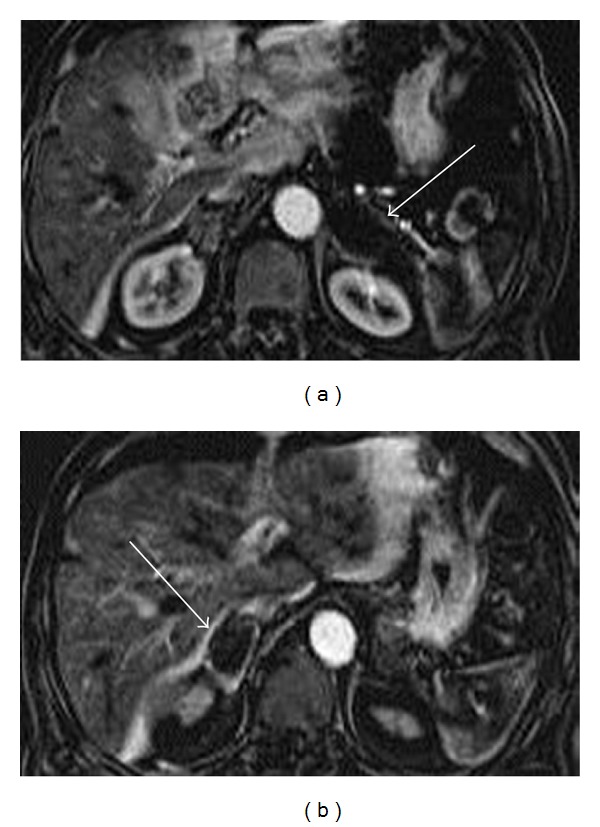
On axial contrast-enhanced subtracted MR images (a), (b) any signal from blood products is removed and the hematoma appears as a signal void (white arrows) because it does not contain enhancing elements, excluding the presence of a hemorrhagic neoplasm.
